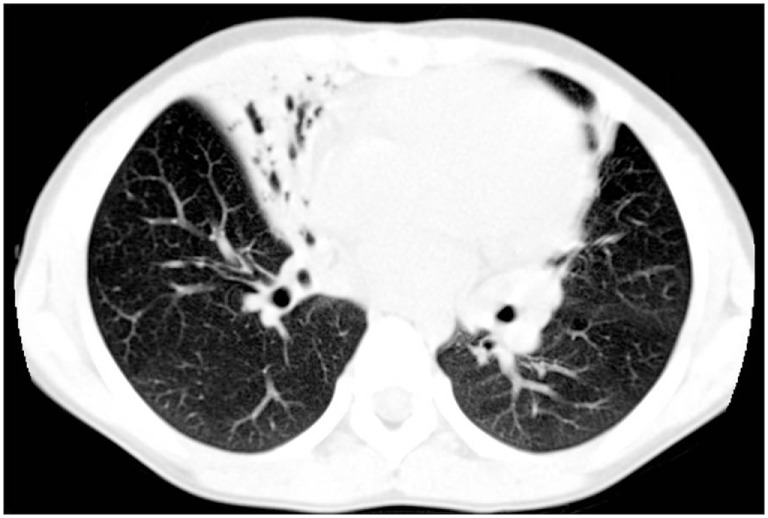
High-resolution computed tomography findings from a 7-year-old girl with primary ciliary dyskinesia. The scan demonstrates an area of consolidation both in the lingula and in the middle lobe, the latter also including bronchiectasis.
